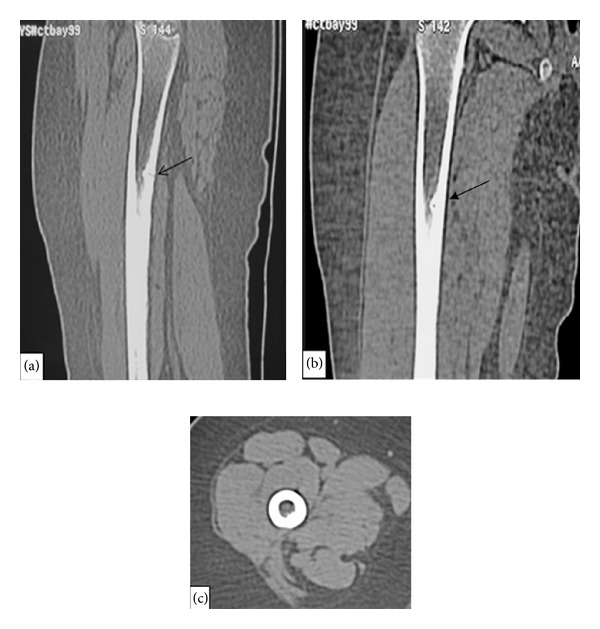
Sagittal (a), coronal (b), and axial (c) reconstructed CT images show a linear defect (arrow) in the medial cortex of the right femur with sclerotic surrounding.
